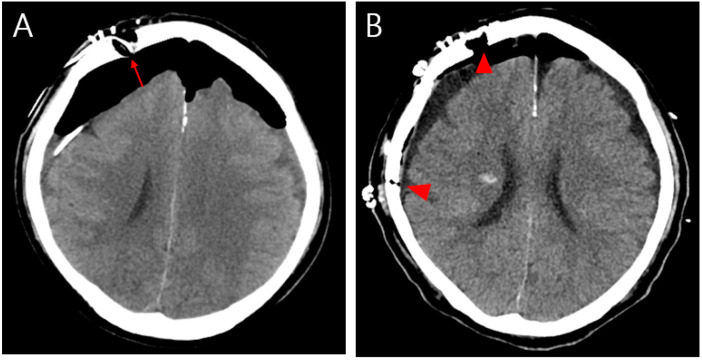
(A) Postoperative brain CT showing persistent intracranial air that compressed the brain parenchyma after burr hole trephination on the right frontal area (arrow). (B) After craniotomy, the CT image of the brain showed an improvement in pneumocephalus upon a reduction in the intracranial air (arrowhead).
